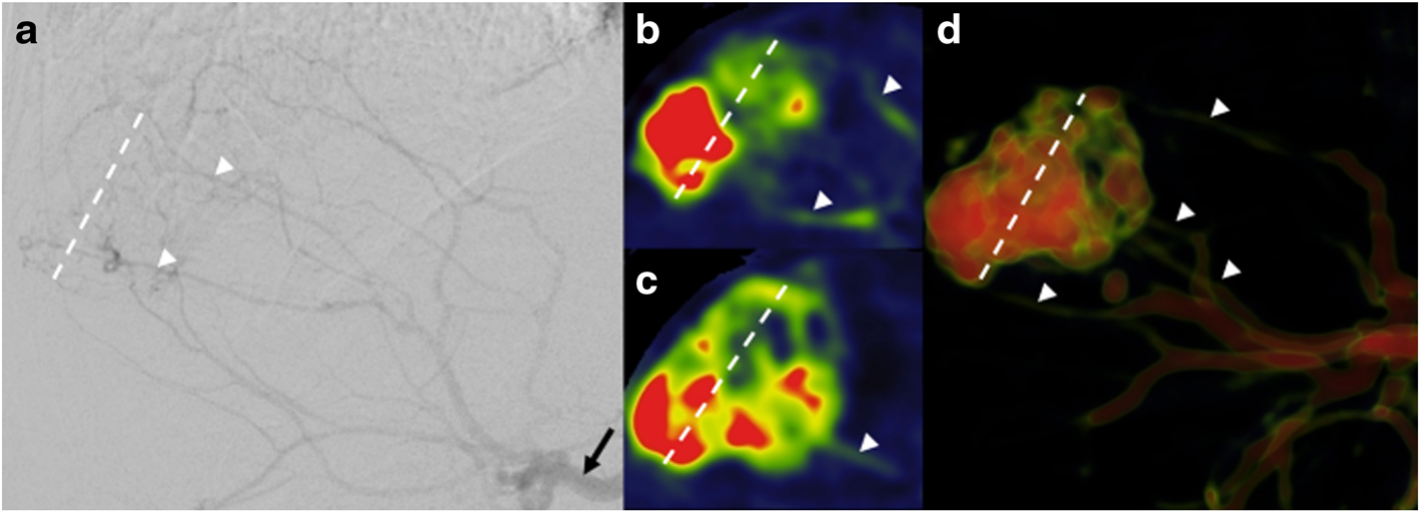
a Angiographic overview shows a hypervascularised HCC lesion in Segment VII (white line) with multiple tumour feeders (white arrows). Extent and location of tumour feeders is confusing. The black arrow marks the right hepatic artery. b–d 2D coronar (b, c) and 3D (d) PBV map reconstructions after C-arm CT acquisition facilitates analysis of location and extent of tumour feeding vessels
